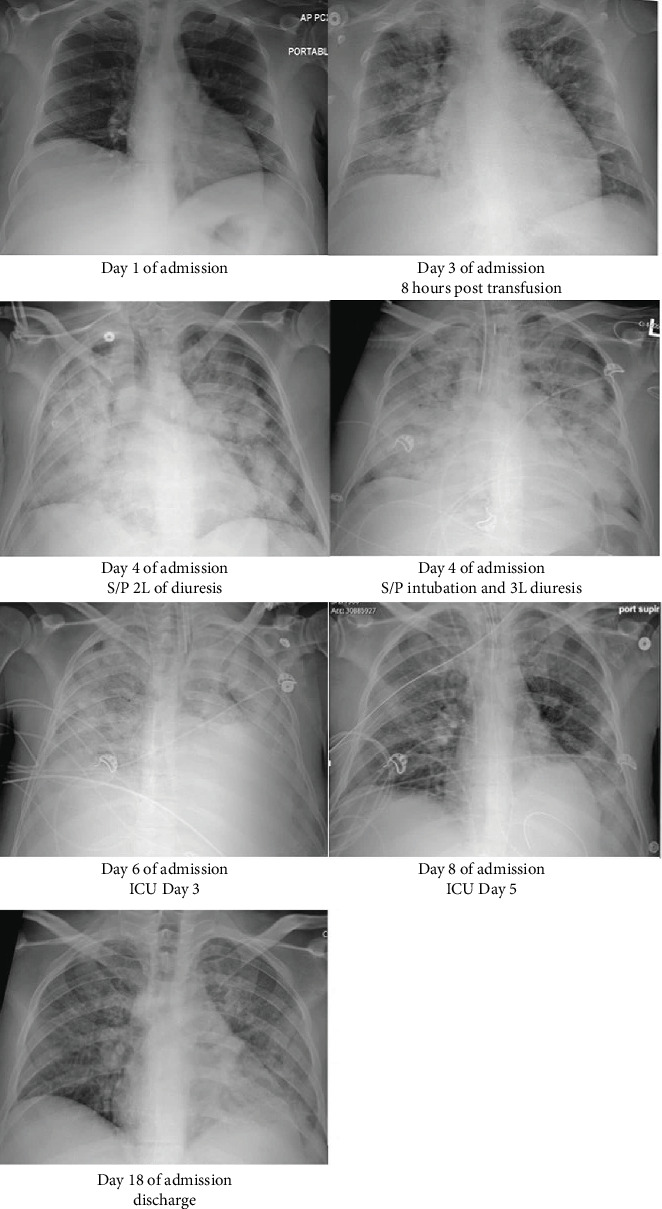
Serial chest x-rays throughout the hospital stay, showing progressive bilateral infiltrates that later improved.
